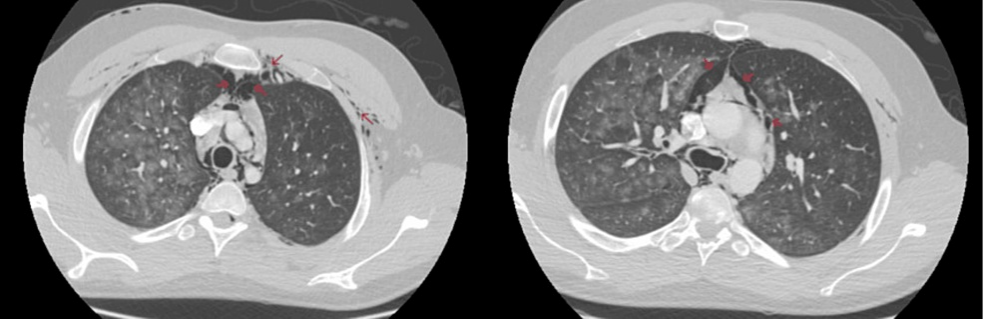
CT of the chest showing areas of air collection within the mediastinum.
